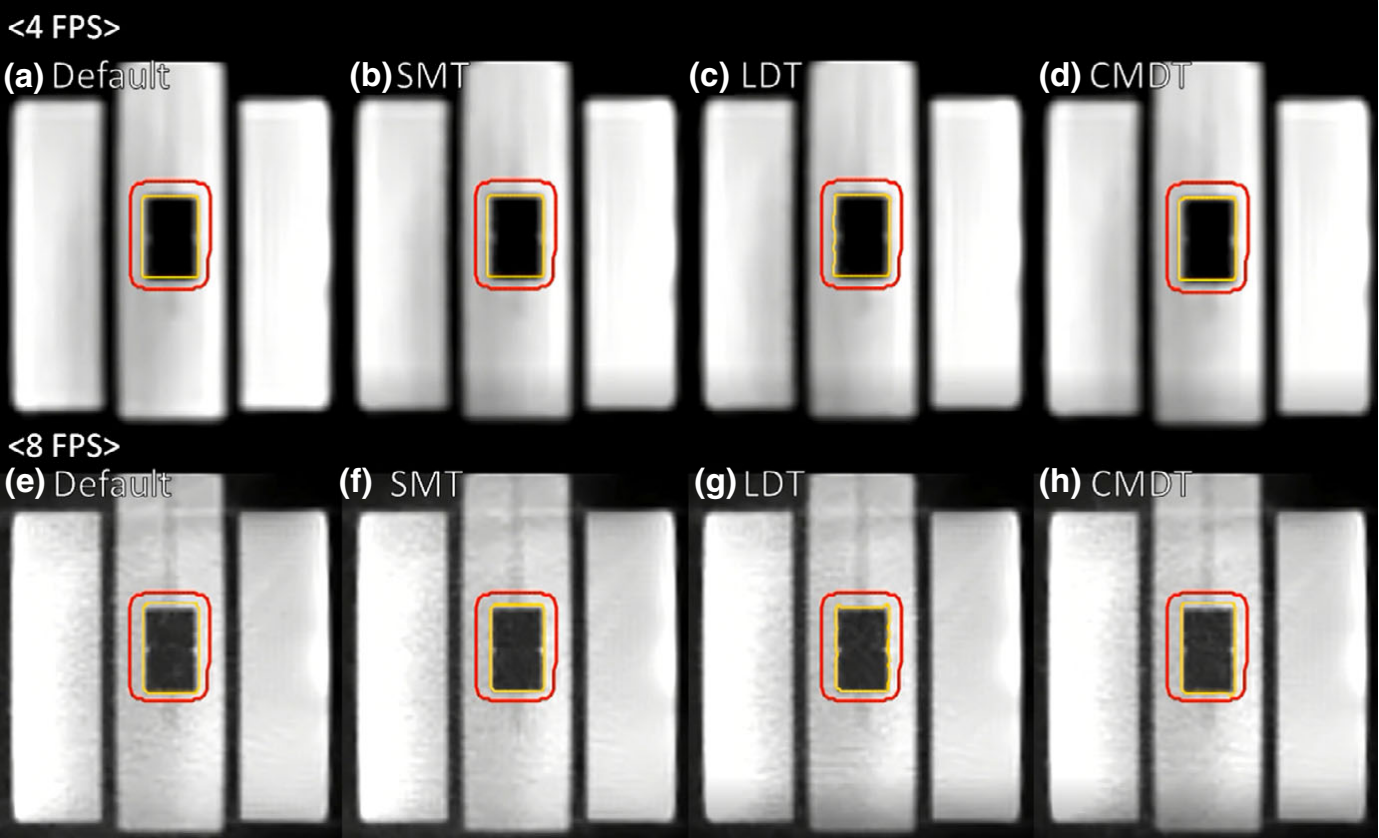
Cine MRI frames from 4 FPS (a–d) and 8 FPS (e–h) acquisitions with the default (a and e), small mobile targets (b and f), large deforming targets (c and g), and complex mobile and deforming targets (d and h) tracking algorithms.
